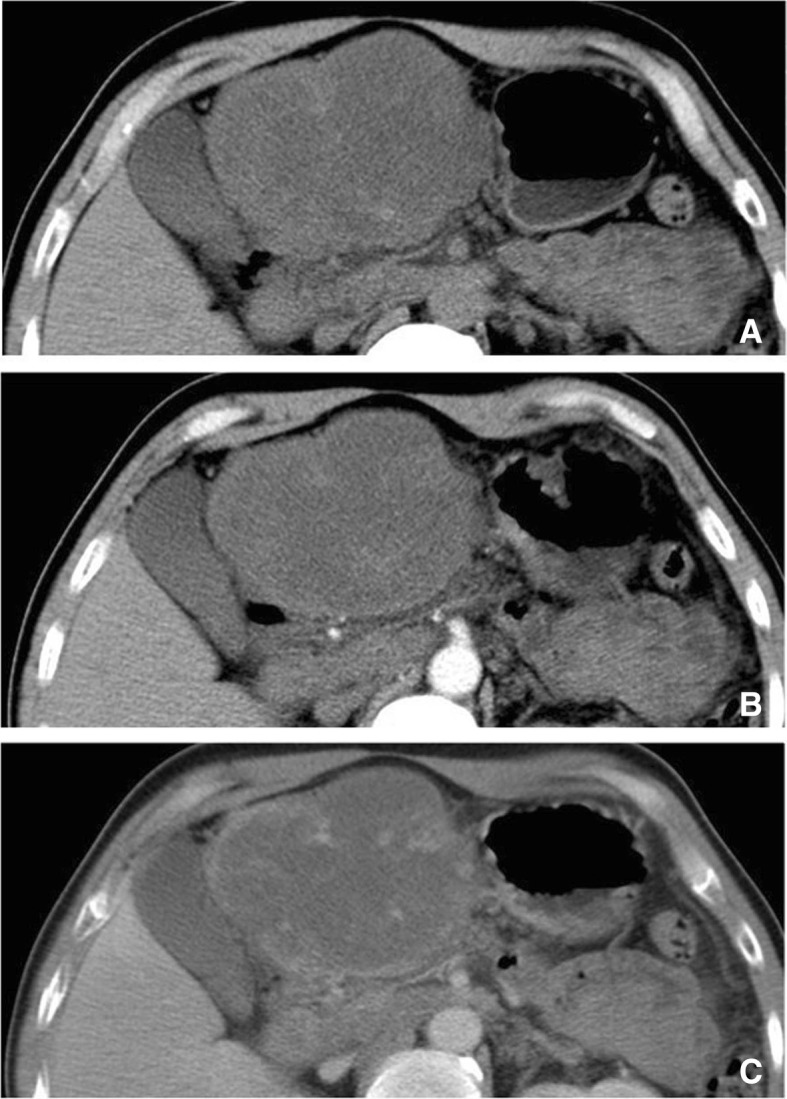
LGMS of the right partes iliaca. X-ray revealed an irregular area of ossification above the right greater trochanter of the femur
